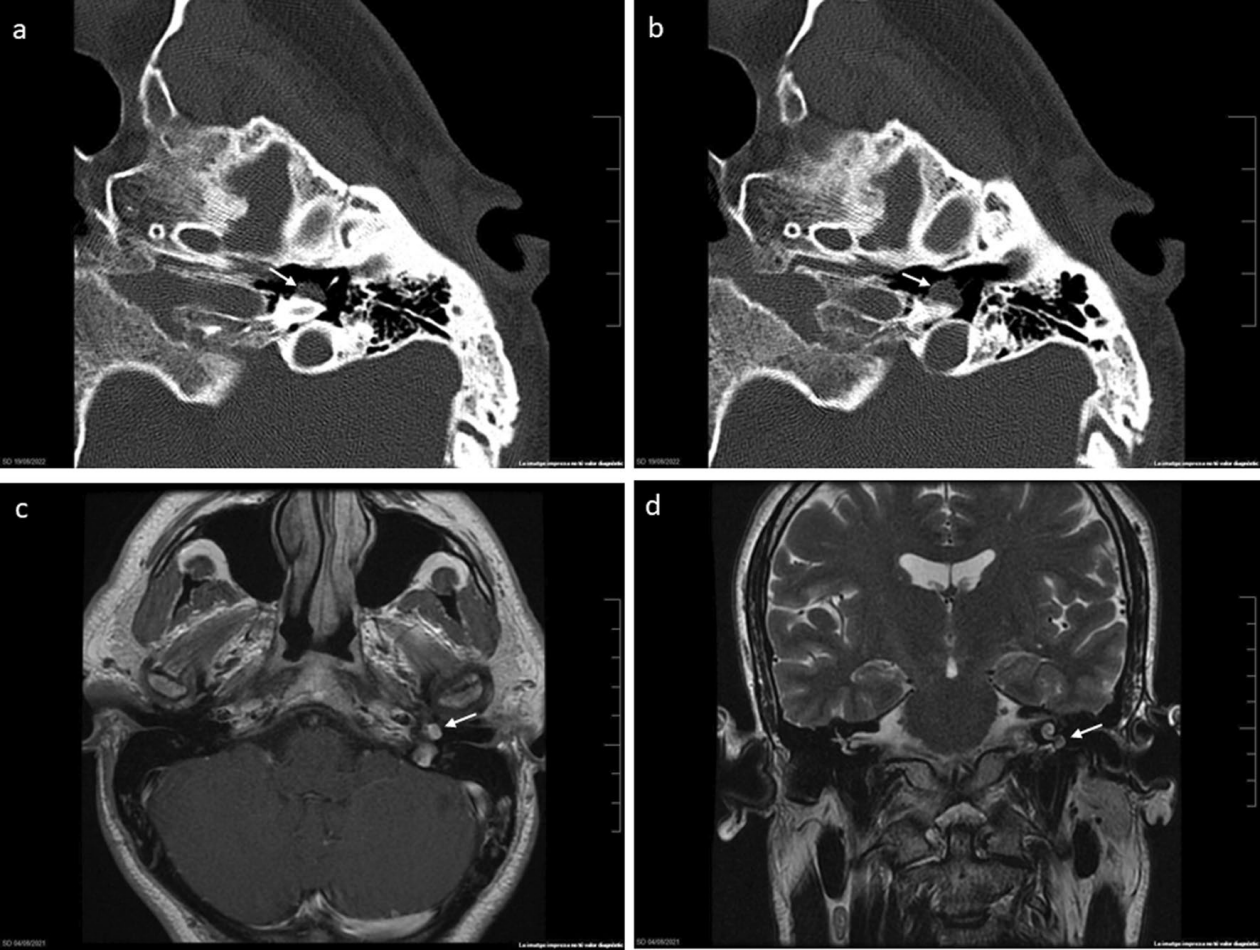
Axial CT scan images showing round lesion located on the promontory (a, b); Same lesion on axial MRI T1 with contrast (c); and coronal MRI T2 sequences
